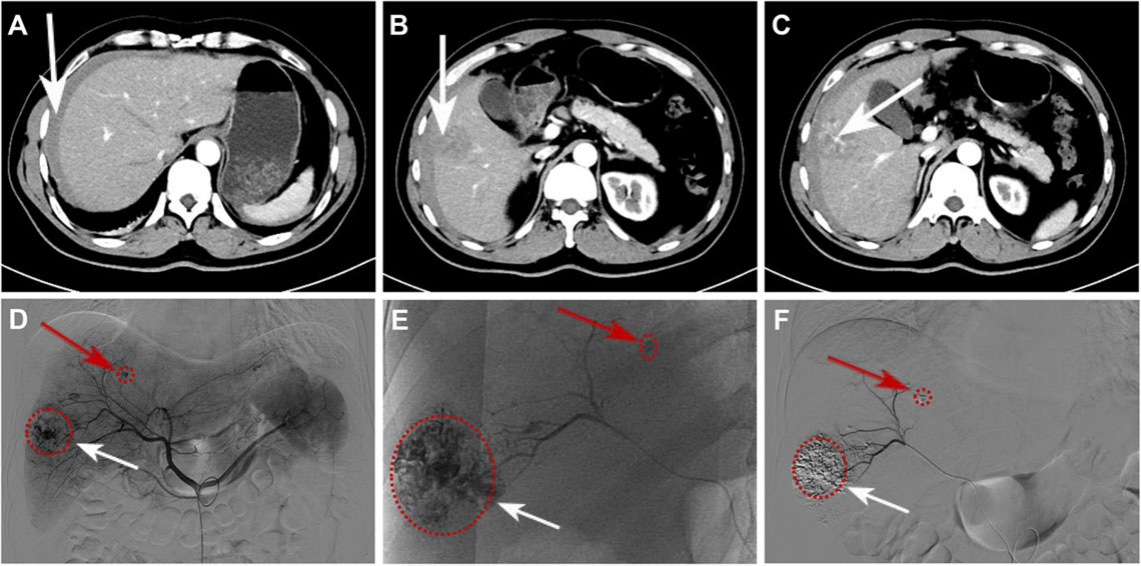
The computed tomography and TAE findings. (A–C) The right lobe of the liver was observed to have a tumor measuring 4.1 × 3.5 cm^2^ in diameter, and bloody ascites was also observed. The situation with the DSA angiography and SHIFT-ICG embolization: (D) before embolization, (E) during embolization and (F) after embolization. White arrow: primary lesions, red arrow: small metastatic lesion.
